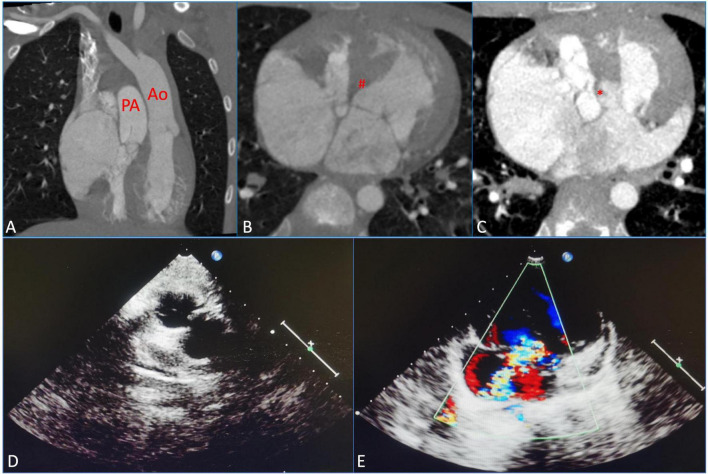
Preoperative measurements using computed tomography, angiography, and echocardiography were assessed before the surgical repair. (A) Congenitally corrected transposition of the great arteries (ccTGAs). (B) Membranous ventricular septal aneurysm (MVSA). (#) Represents MVSA. (C) Atrial septal defect (ASD). (*) Represents ASD. (D) Severe pulmonary stenosis (PS). (E) Severe tricuspid regurgitation (TR). PA, pulmonary artery; Ao, aorta.
